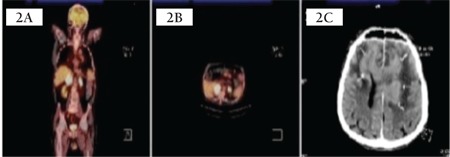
The images of PET-CT and Cranial MR
